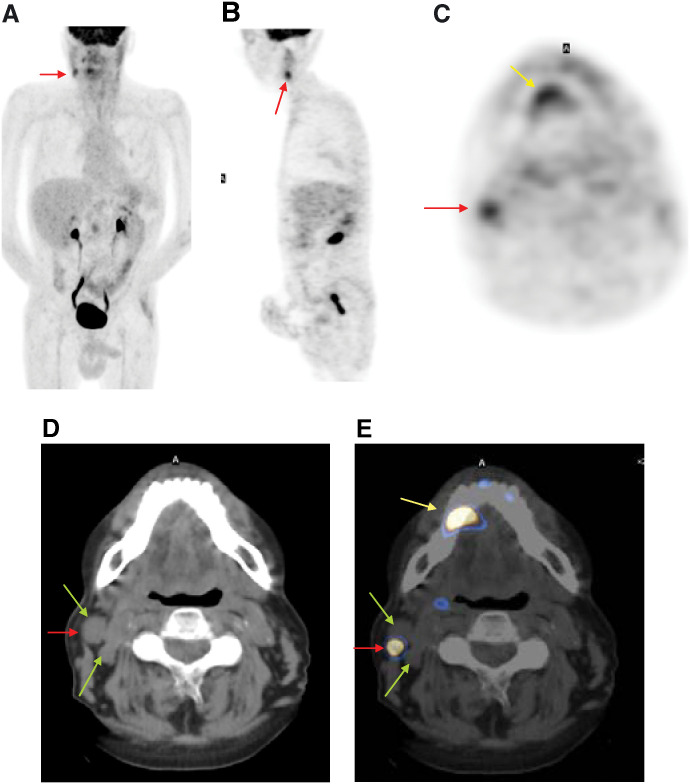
A 50-year-old male with squamous cell carcinoma of the tongue. (A) Multiple intensity projection ^18^F-FDG PET image. (B) Sagittal and (C) transaxial images show abnormal uptake in the right cervical region and right premolar area; dental pathology is present (yellow arrow). (D) CT transaxial section reveals level II enlarged lymph nodes. (E) Fused ^18^F-FDG PET/CT transaxial section at the same level reveals that one lymph node is FDG positive (red arrow), while the other nodes shows no avidity for FDG (green arrows). The fused images clearly localised the exact site of involvement.
